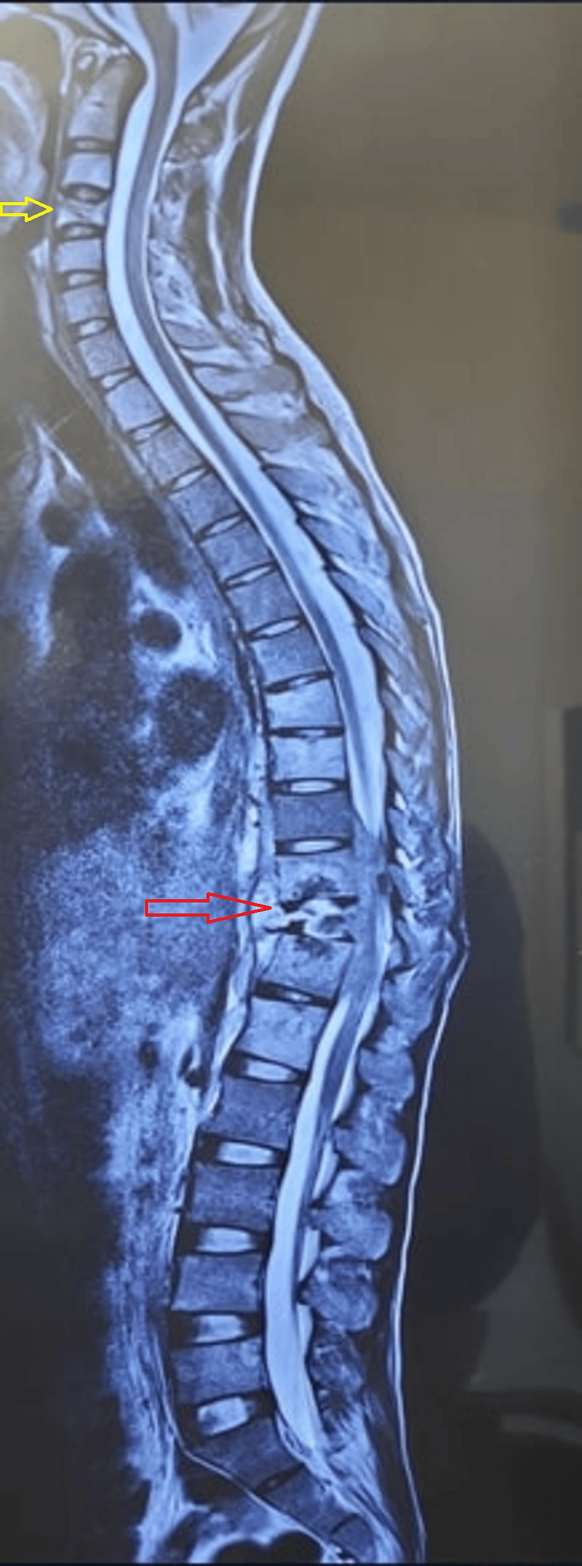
Whole spine screening T2W MRI of a patient who presented with axial neck pain as the chief complaint. MRI revealed multifocal lesions at C4, D7-8, and D10-12. Rather than taking a biopsy sample from C4 (yellow arrow), a transpedicular biopsy was done from the D12 vertebral body and D11-12 disc space (red arrow). This approach was more easy, convenient, and risk-free TB: tuberculosis; MRI: magnetic resonance imaging
